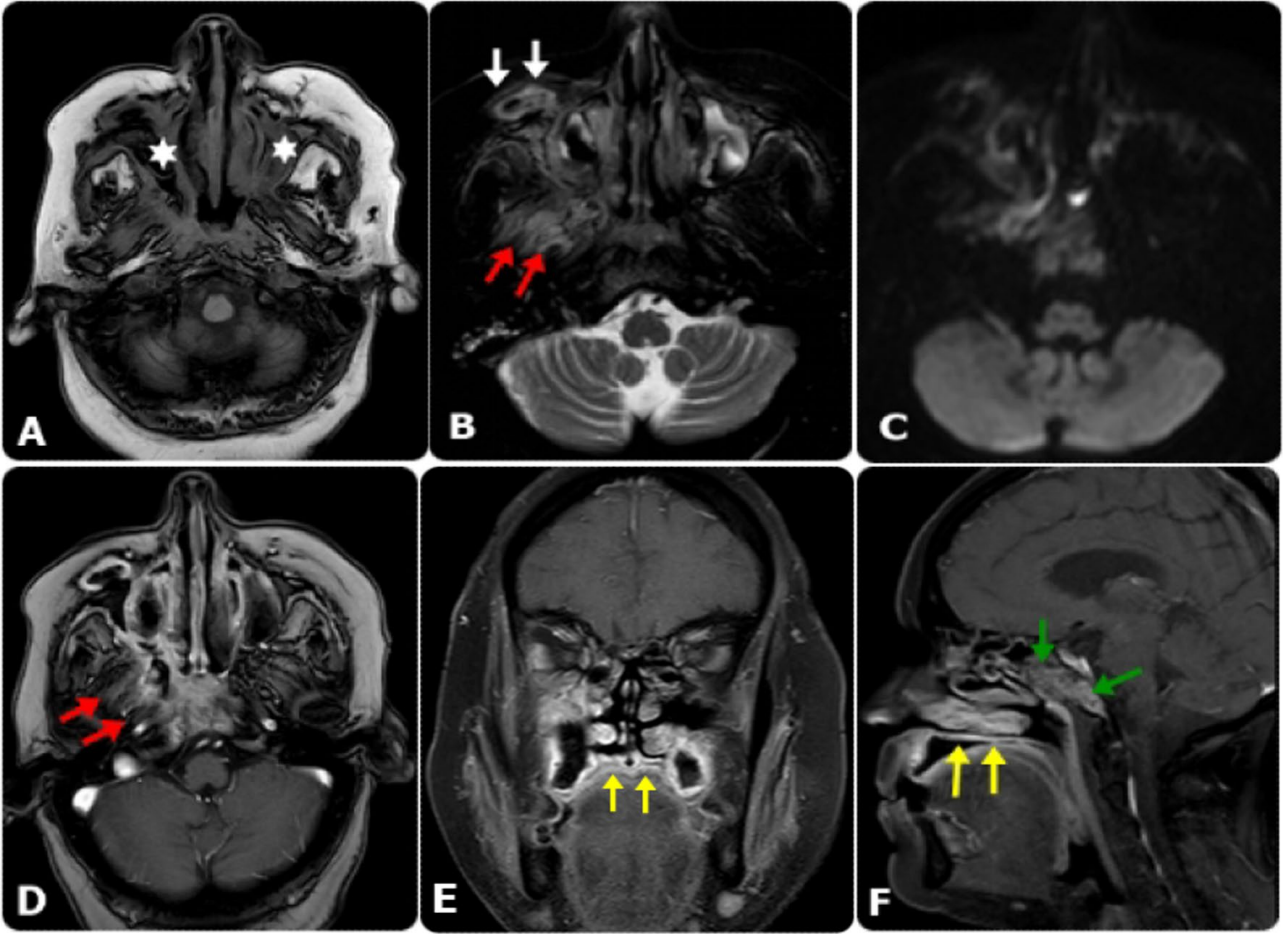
MRI findings in diabetic female patient, 56 years old, presented mainly with Rt. Maxillary swelling, following COVID-19. A Axial T1W, B and Axial T2-SPIR images showed bilateral maxillary sinusitis (Asterisks), with mixed signal intensity within representing heterogeneous pattern of affection, associated with detected Rt. Pre-maxillary small collection (white arrows), presented restricted diffusion on DWI at b-value 1000 (C) and showing peripheral pattern of enhancement on post-contrast axial T1W image (D). The edema and inflammatory process seen extending to the Rt. retro-maxillary region (Red arrows). Coronal (E) and sagittal (F) post-contrast fat-saturated T1W images revealed extension of the edema and inflammatory process to the hard palate (yellow arrows) and to the base of the skull (Green arrows)
